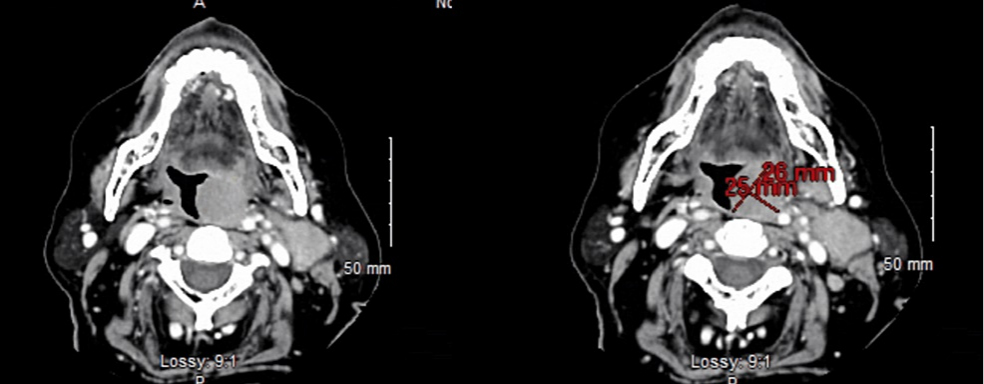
CT maxillofacial with IV contrast depicting a 25 mm x 26 mm oropharyngeal mass
